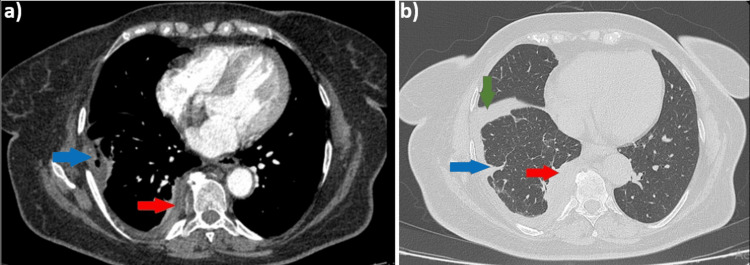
Contrast Chest CT scan, (a) mediastinal window, axial plane; (b) lung window, axial plane, describing paravertebral pleural thickening, pleural effusion, and fluid accumulation in the right fissure.
